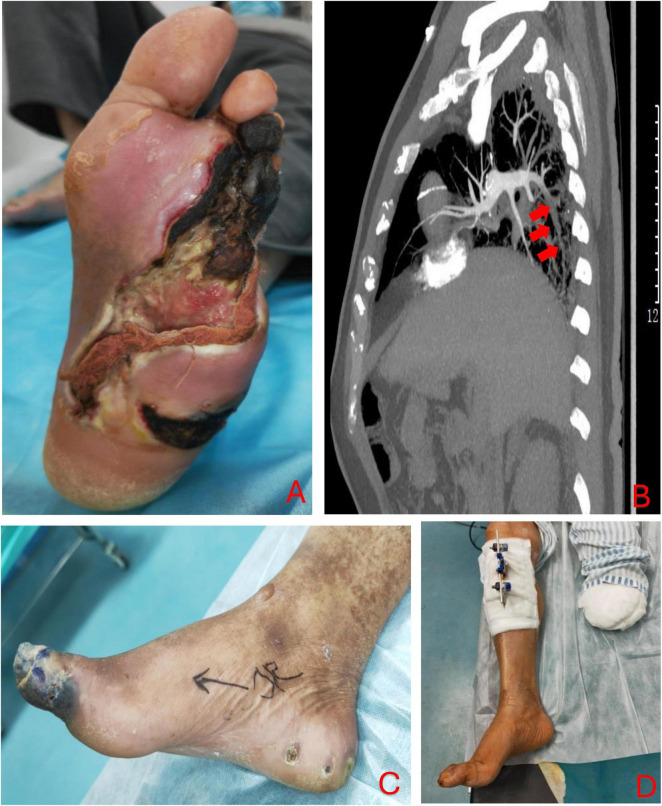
The initial presentation of left foot at the time of admission (A); CTA scan revealed emboli in the posterior basal branch of pulmonary artery to right lower lobe (as marked by the red arrows) (B); acute limb ischemia in right lower limb despite on anticoagulation therapy (C). Transtibial transport (TTT) in the right lower limb was done and the tissue perfusion was restored which reversed the hallux necrosis (D).
